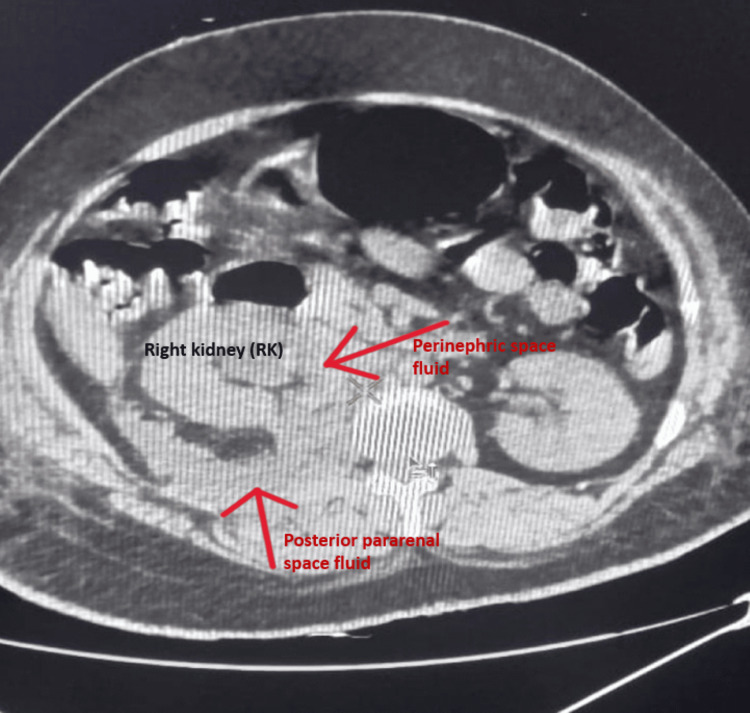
Axial CT abdomen demonstrating retroperitoneal fluid collections in the perinephric and posterior pararenal spaces Axial noncontrast CT abdomen demonstrating retroperitoneal fluid collections consistent with urinary extravasation. The upper arrow indicates fluid within the right perinephric space (between Gerota's fascia and the renal capsule), consistent with a urinoma. The lower arrow indicates extension of fluid into the posterior pararenal space. The right kidney is visible to the right of the midline with surrounding fat stranding CT: computed tomography
